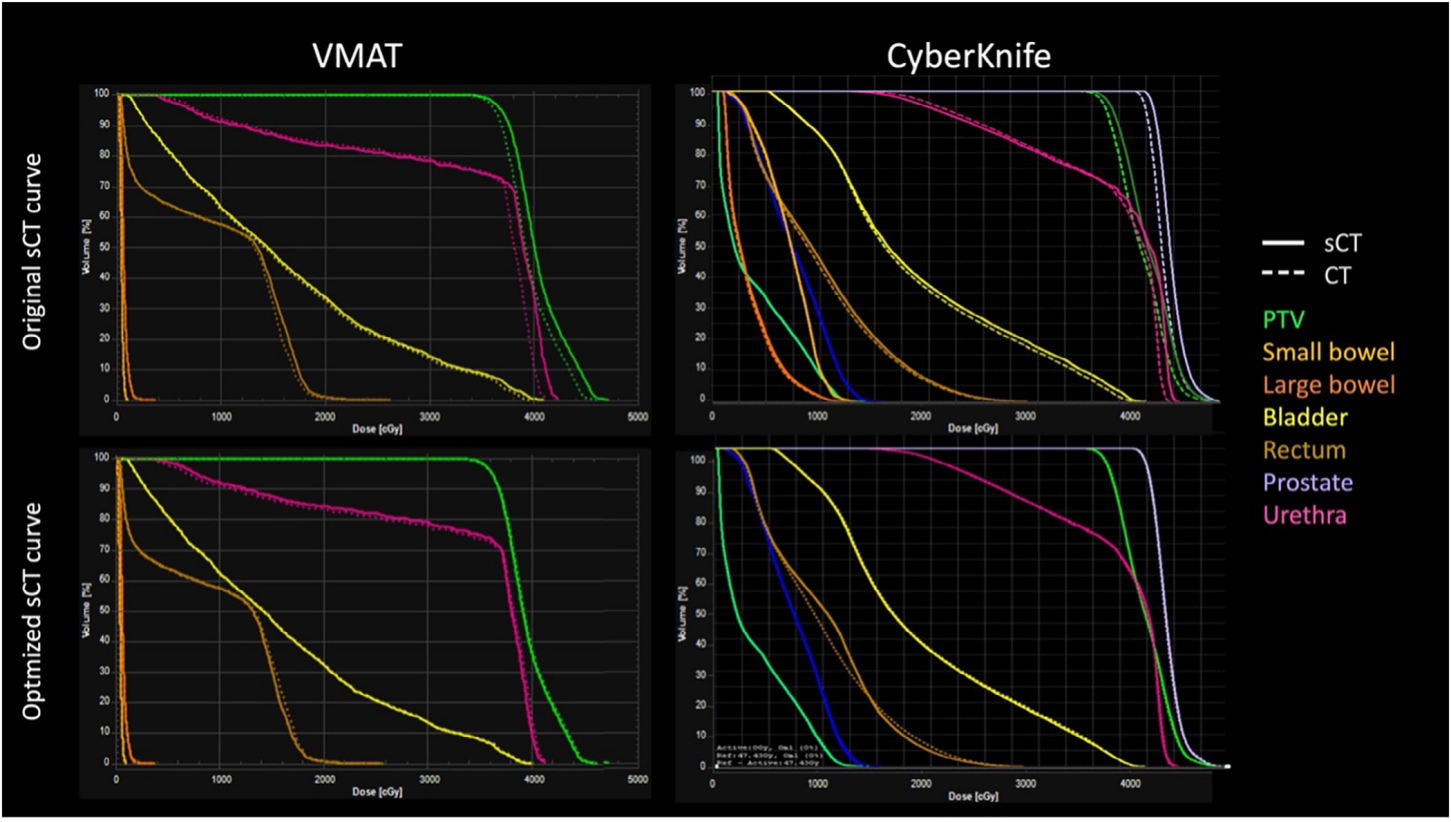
Example DVH plots in sCT (solid line) versus CT (dashed line) datasets demonstrating the systematic discrepancies when using the original CT calibration curve (top row) which are resolved when implementing the optimized CT calibration curve (bottom row) for VMAT (left column) and CyberKnife (right column) treatment plans. CT, computed tomography; DVH, dose‐volume‐histogram; sCT, synthetic CT; VMAT, volumetric modulated arc therapy.
